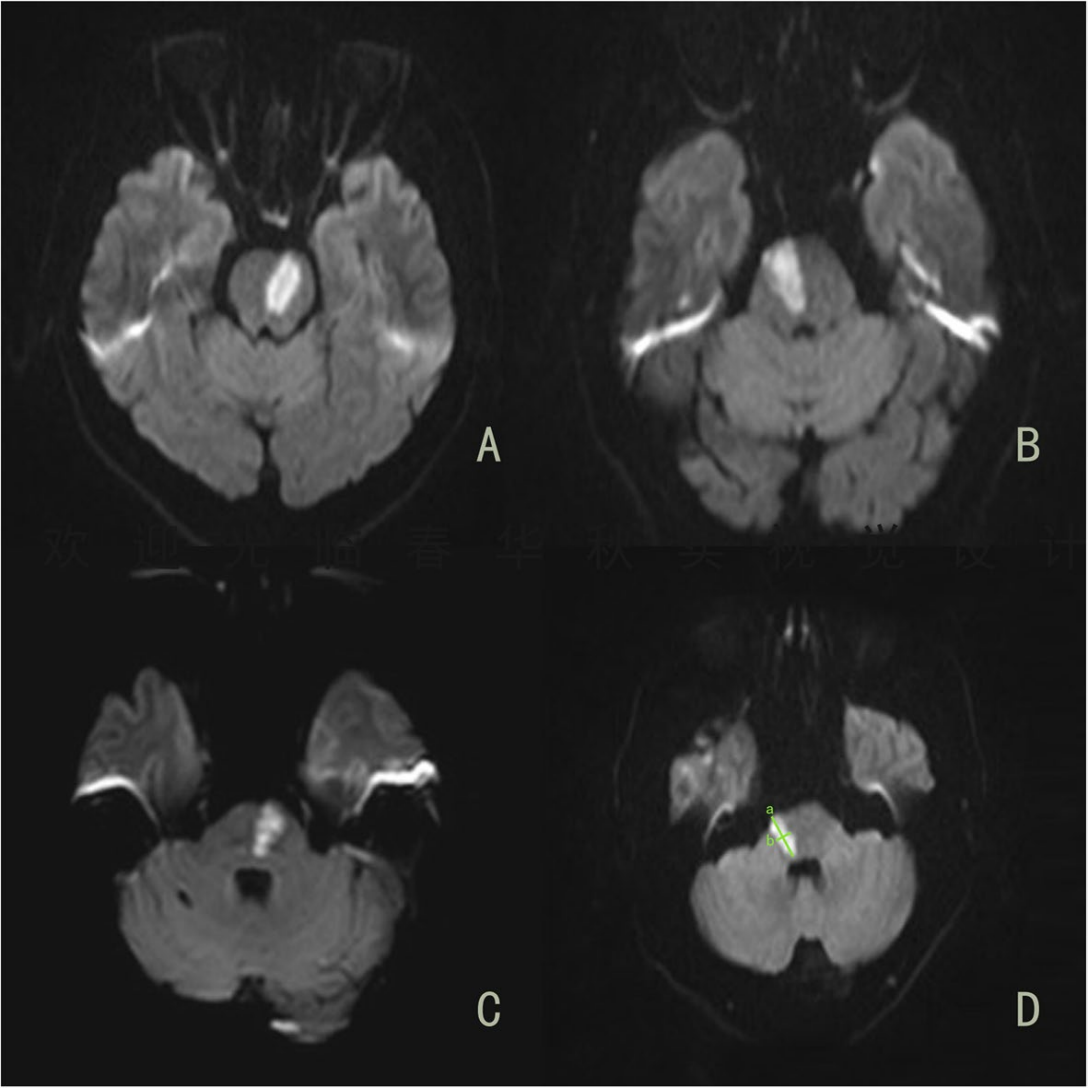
(A): upper pons; (B): middle pons; (C): lower pons; (D): API volume measurement based on DWI: a multiplied by b and then multiplied by the number of layers divided by 2
